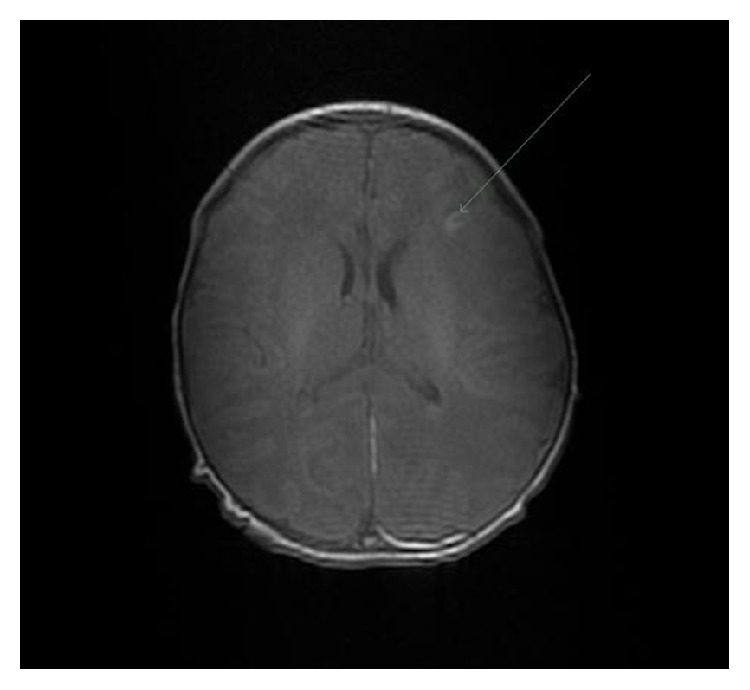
Magnetic resonance imaging demonstrating a 1–1.5 cm focal area of parenchymal abnormality in the left frontal lobe anterolaterally, appearances of which are consistent with infarction.
